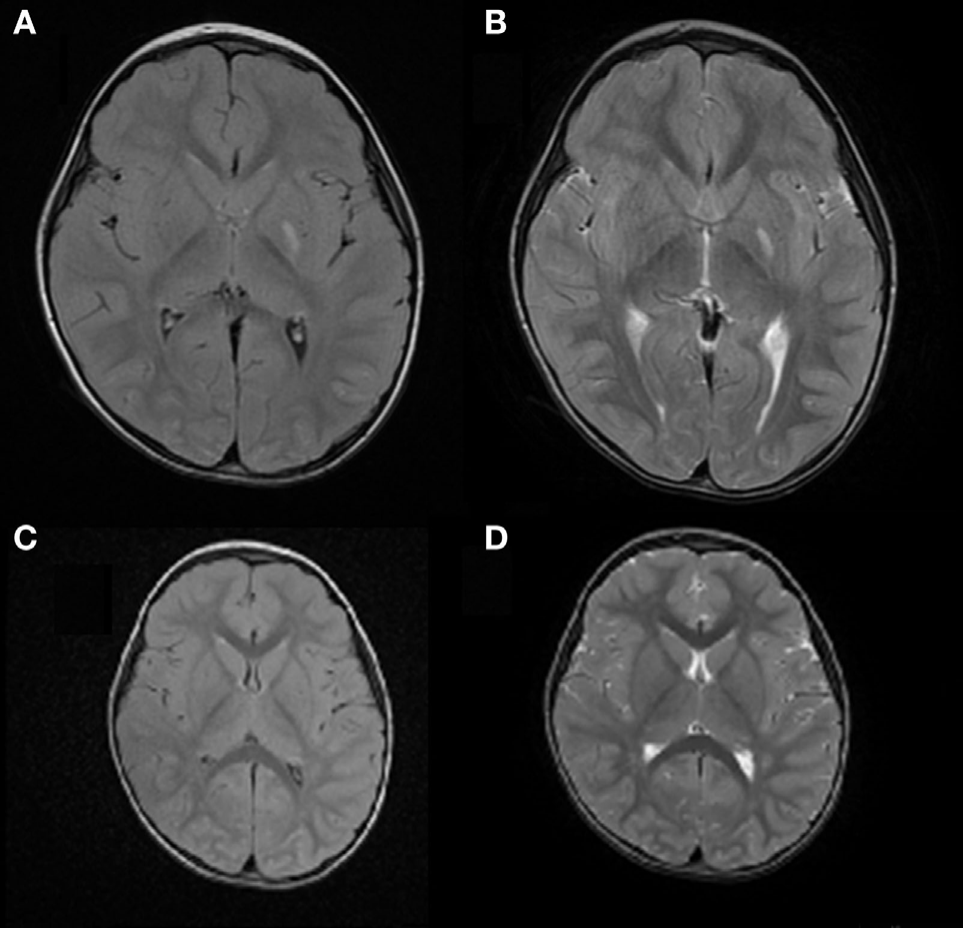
Case 1: (A) axial FLAIR image and (B) axial T2 weighted image, showing ischemic lesion involving the left lentiform nucleus and posterior limb of the internal capsule. (C) Axial FLAIR image and (D) axial T2 weighted image showing resolution of the left lentiform nucleus lesion and reduction of the posterior limb involvement in the internal capsule.
